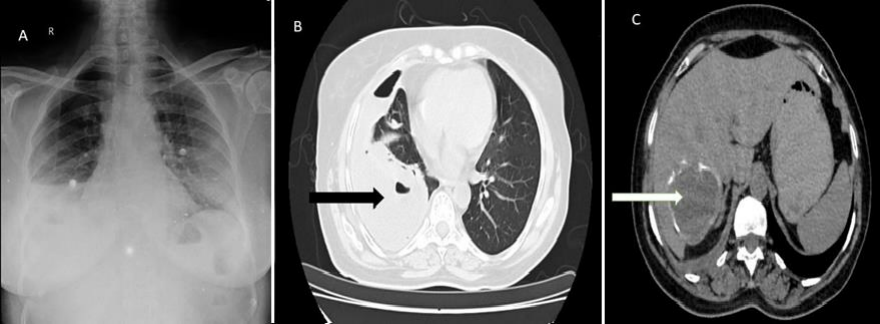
A) chest X-ray of the patient showing right pleural effusion; B) computed tomography (CT) of the thorax with a black arrow showing intrapulmonary hydatid cyst with pleural extension; C) CT of the abdomen with a white arrow showing hepatic hydatid cyst
